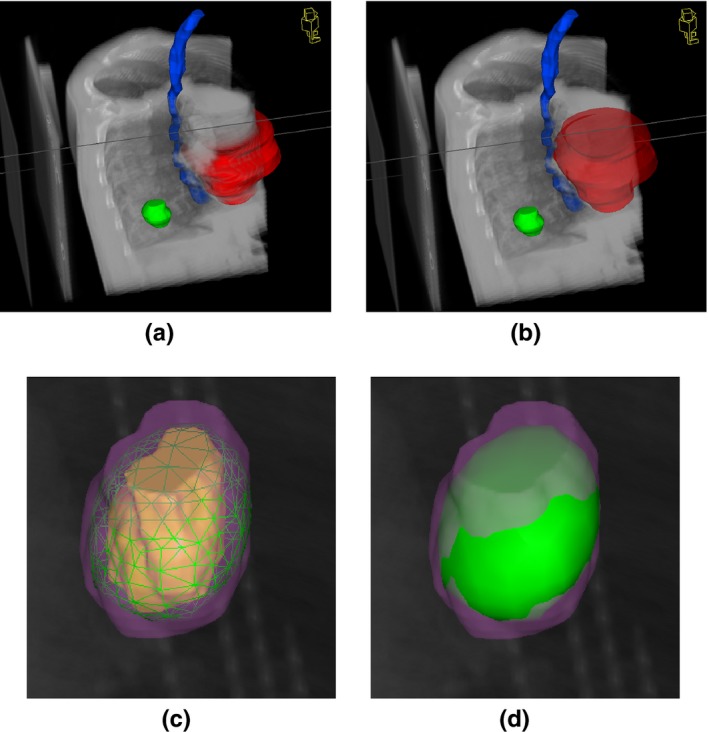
Using C‐TPS for task T.1 and T.2: Contours are visualized together with the planning CT. Clipping is applied, however, only the CT is affected. The ITV is depicted in green, the heart in red and the esophagus in blue. In (b), the heart is made slightly transparent. When compared to (a), the volume covering the heart is not shown correctly anymore. C‐TPS does not preserve the depth information of the heart when made transparent. Using C‐TPS for task T.3: Green is the 37.5 Gy iso‐dose. The planning target volume is depicted in violet, and the ITV in yellow. In (c), the iso‐dose surface is visualized as mesh, and as solid surface in (d).
